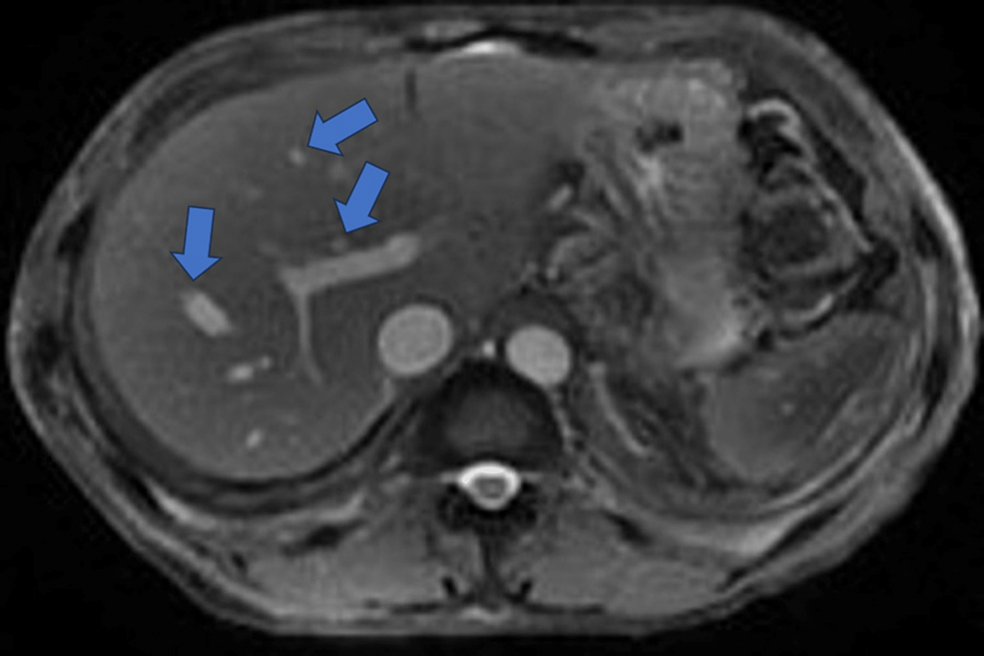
MRI abdomen with and without IV contrast showing hepatic steatosis (blue arrows)
